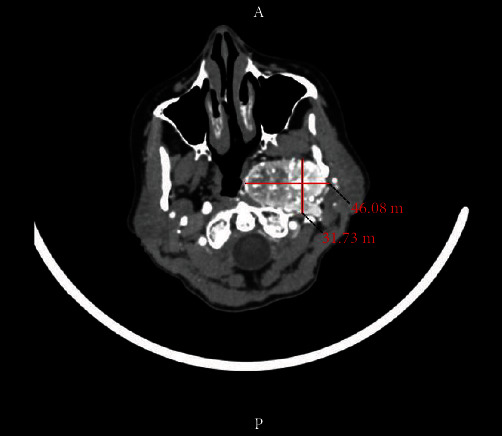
CT neck showing encasement and marked narrowing of the left cervical internal carotid artery.
